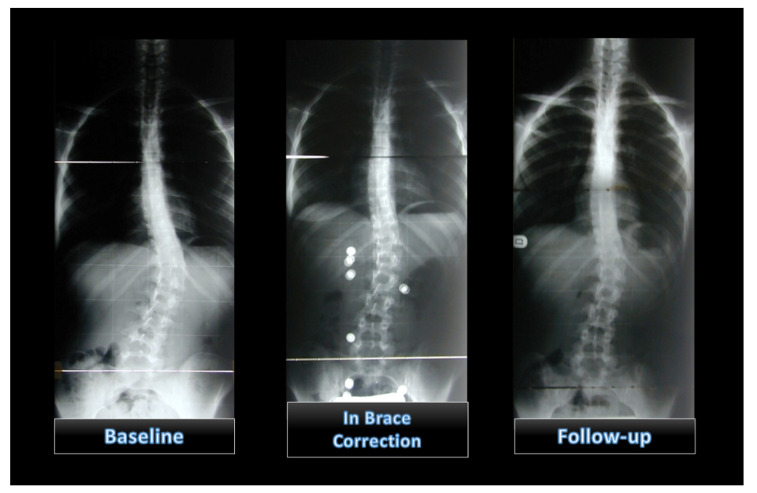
Patient X-rays that show changes over time.
